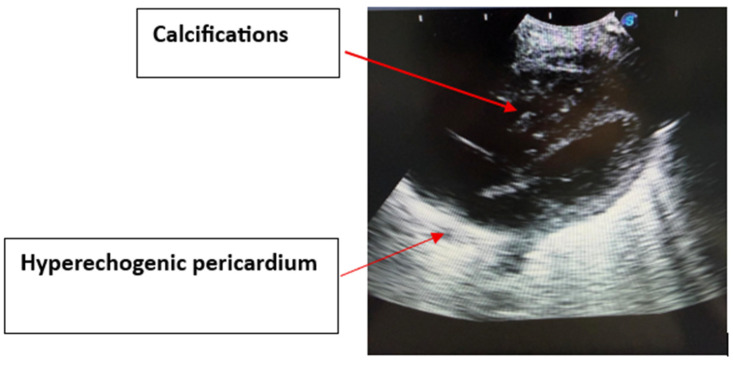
Echocardiography PLAX view.
